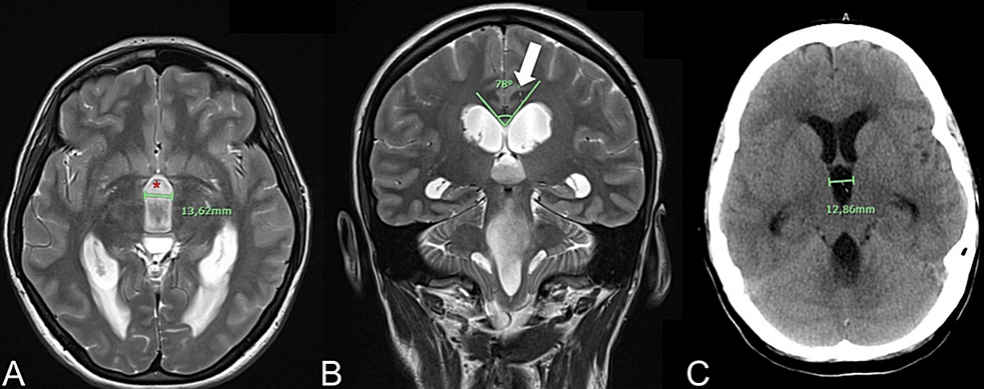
Radiological findings of Case 2 (A, B) MRI performed at the hospital admission: the axial and coronal images show ventriculomegaly with enlargement of third ventricle (red asterisk) and reduction of callosal angle (78°, white arrow). (C) Head CT scan performed at the time of neurological impairment, with negative COVID-19 test: no volume alteration of the ventricular system.
